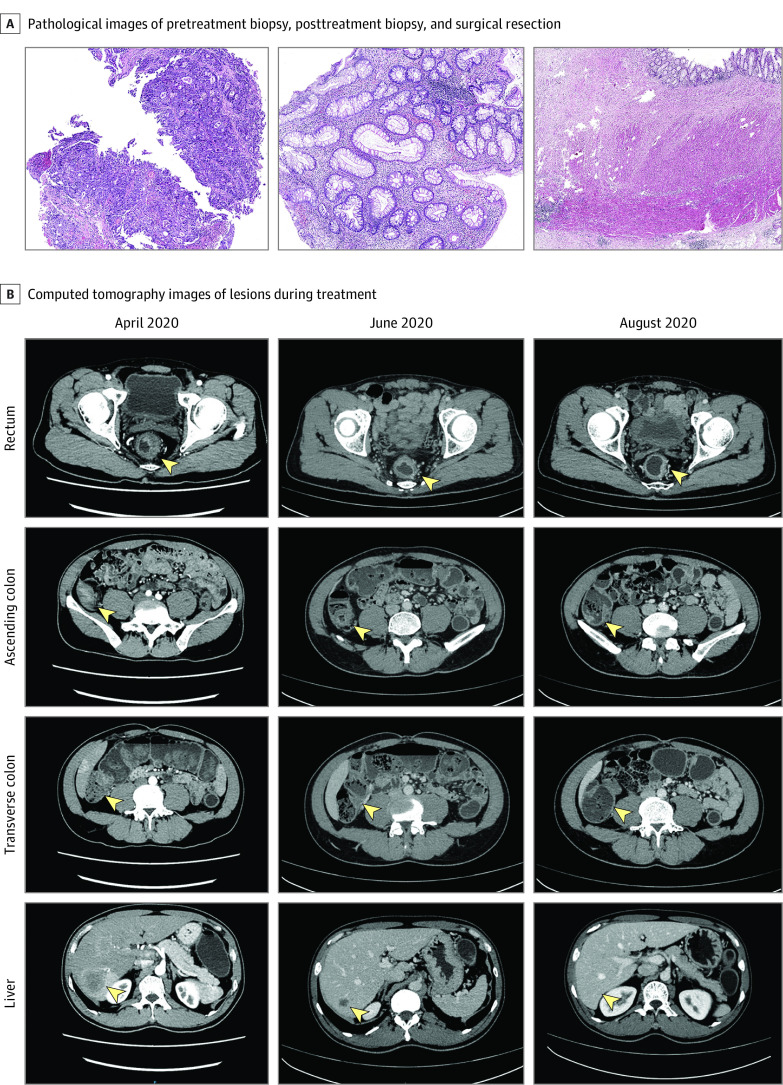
Treatment Characteristics of a Patient With Synchronous Multiple Primary Colorectal Cancer With Liver Metastases A, Pathological images of pretreatment biopsy (left, magnification, 60×), posttreatment biopsy (middle, magnification, 60×), and surgical resection (right, magnification, 20×) are shown. B, Computed tomography images of each lesion (arrowheads) during treatment are shown.
